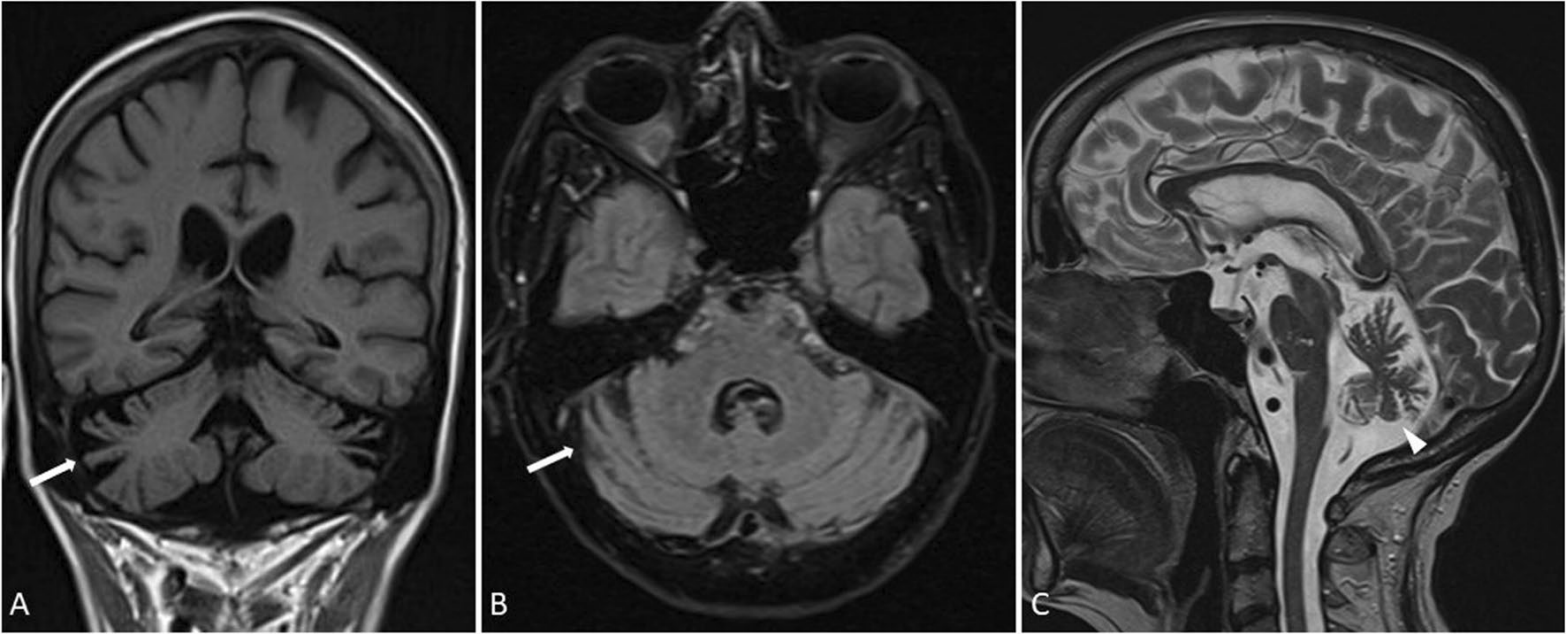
MR image of the patient shows atrophy of cerebellar hemispheres (arrows) in Axial T2-FLAIR images a and coronal T1-weighted images b and vermis atrophy (arrowhead) in sagittal T2-weighted images (c)
